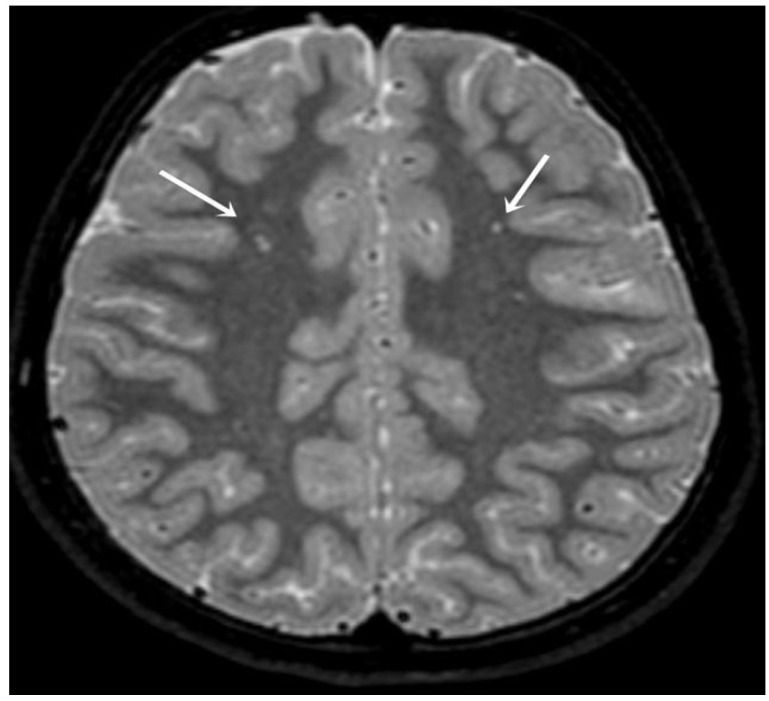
Bilateral subcortical hyperintensities (white arrows) on an axial plane FLAIR image from a 10-year-old girl exposed to frequent migraine attacks.
